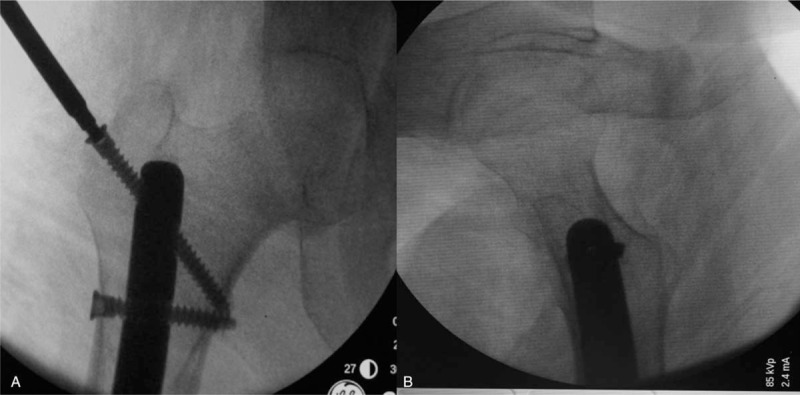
Successful nailing by navigator under intraoperative x-ray. A, Anterior-posterior x-ray of femur during operation. B, Medial-lateral x-ray of femur during operation.
